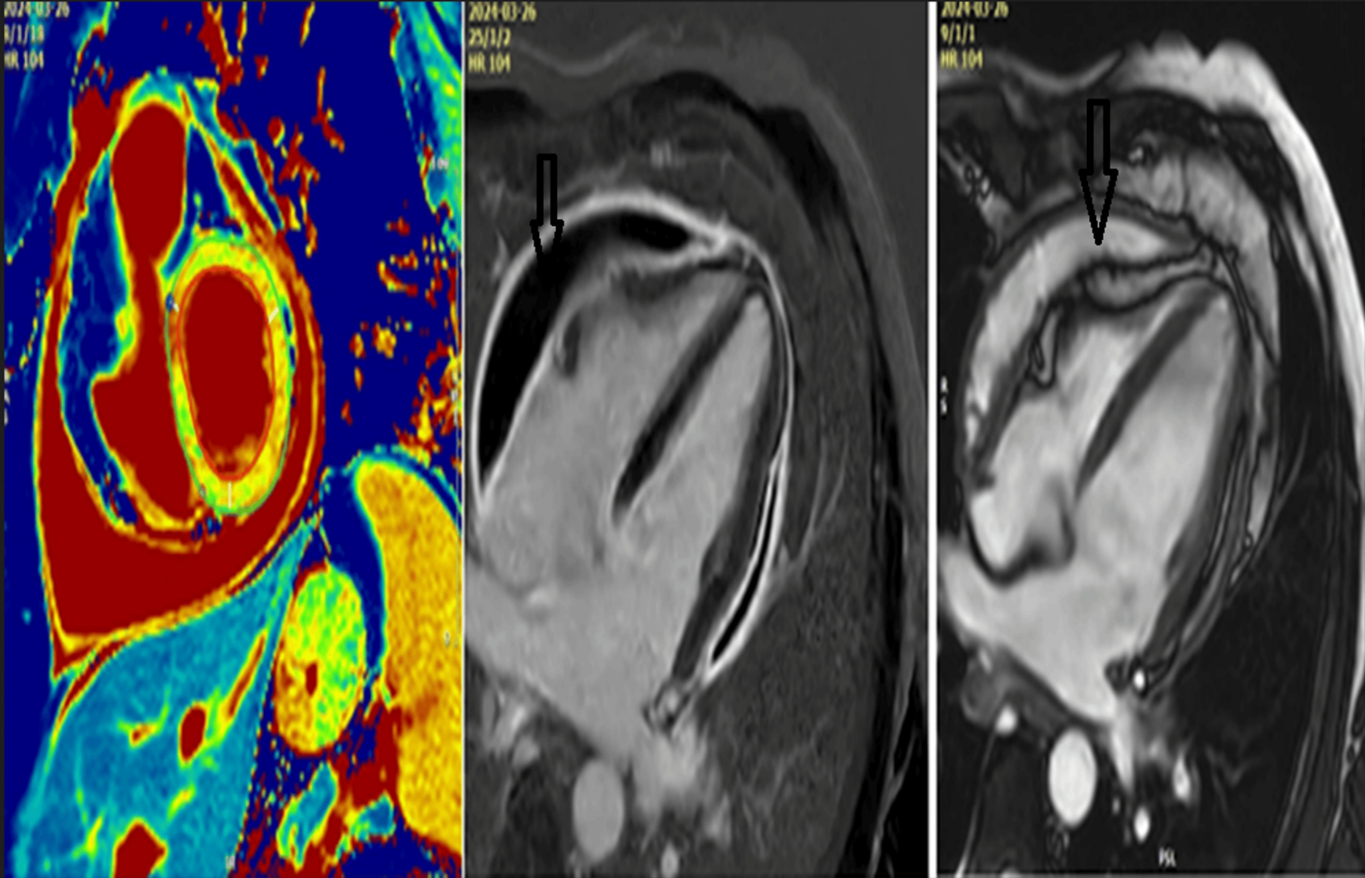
Cardiac MRI Cardiac MRI confirms the diagnosis of effusive constrictive pericarditis
